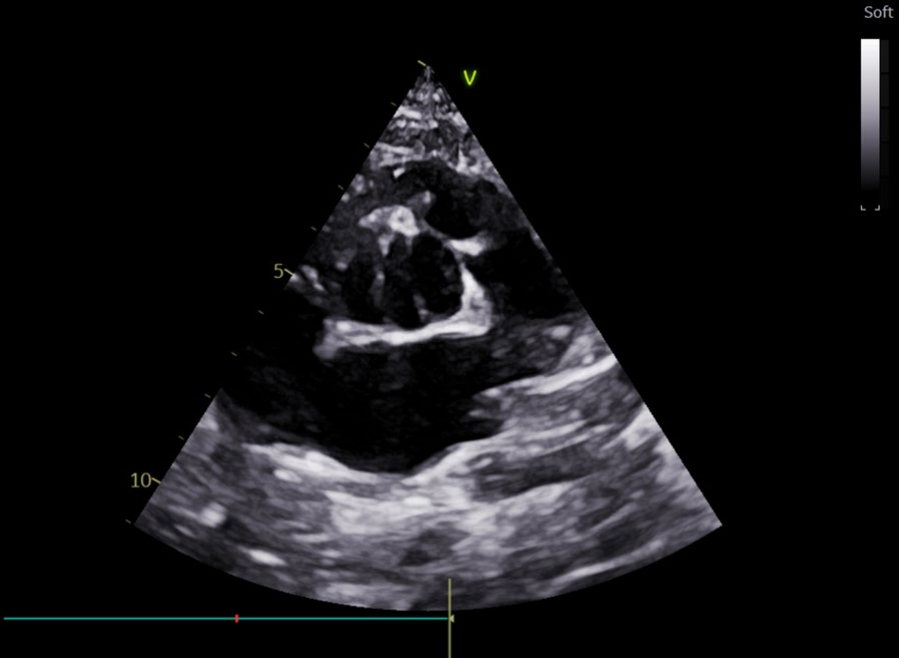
Parasternal short axis view at level of aortic valve showing bicuspid aortic valve without raphe in open position
